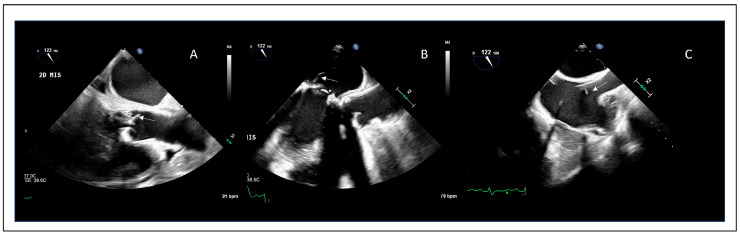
Transesophageal echocardiographic findings across different infective endocarditis setting. (A) Native valve infective endocarditis. Mid-esophageal transesophageal echocardiographic view of the native aortic valve showing degenerative calcific changes of the cusps. A thin, filiform echodense structure is visible on the non-coronary cusp (arrow), consistent with a valvular vegetation. (B) Prosthetic valve endocarditis. Mid-esophageal transesophageal echocardiographic view of a mitral bioprosthesis demonstrating a filiform echodense formation measuring approximately 10 mm, attached to the prosthetic leaflet (arrow), compatible with prosthetic valve involvement in infective endocarditis. (C) Cardiac device-related infective endocarditis. Bicaval transesophageal echocardiographic view showing a filiform echodense structure adherent to an intracardiac pacing lead (arrow), consistent with a vegetation attached to the lead.
